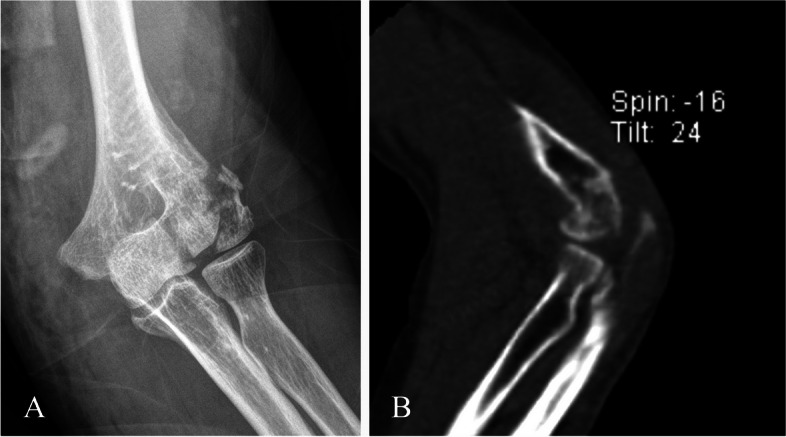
A type IIIB capitellum fracture in a 61-year-old woman who fell on an outstretched hand. A Preoperative anteroposterior radiograph and B sagittal computed tomography scan
